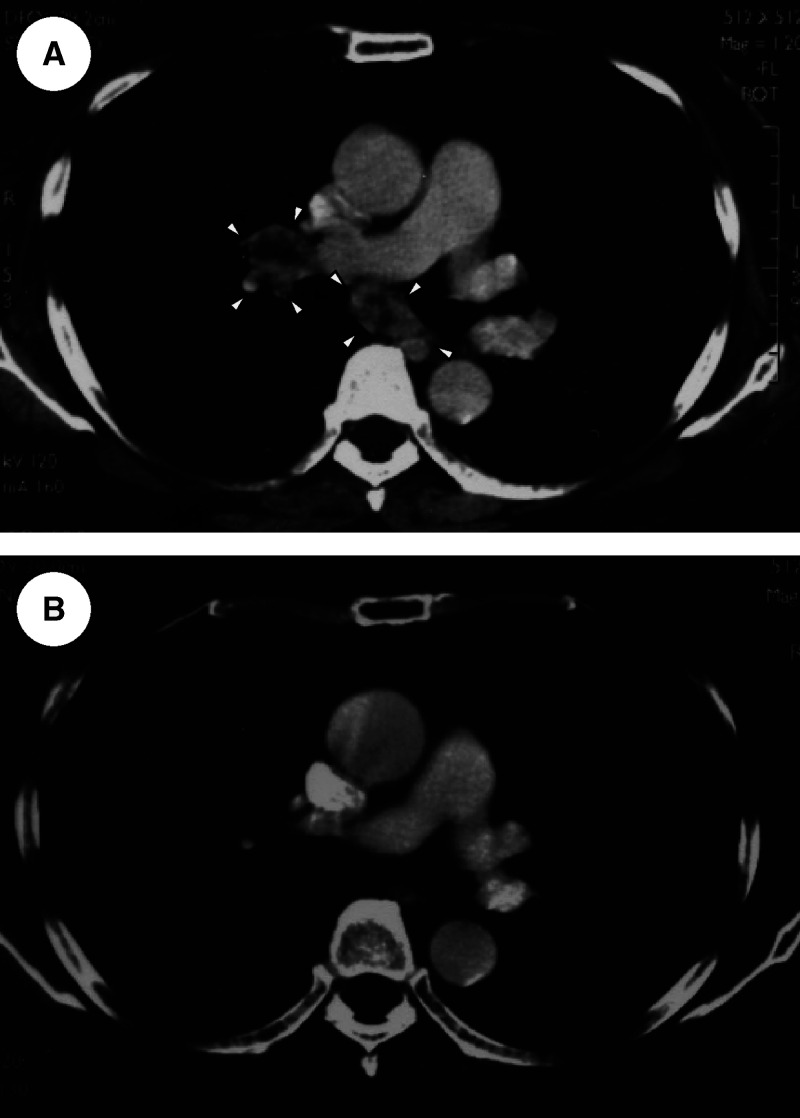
(A) Patient No. 8 chest CT shows mediastinal lymph nodes at diagnosis (arrrowheads). (B) Mediastinal lymph node swelling disappeared after immunotherapy (CR).
